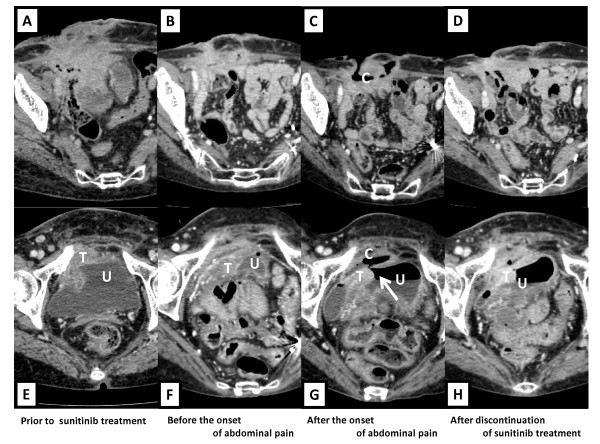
CT findings. U, urinary bladder; T, tumor (GIST); C, subcutaneous cavity. Tumor was located between abdominal wall (A) and urinary bladder (E) before sunitinib therapy. Abdominal tumor was smaller on day 4 of fourth course of sunitinib (B and F). Vesicocutaneous fistula bridged by subcutaneous cavity at onset of abdominal pain (C and G). Small opening (arrow) at anterior bladder wall. Air collected in urinary bladder. Fistula healed after sunitinib discontinuation (D and H) and subcutaneous cavity became smaller.
